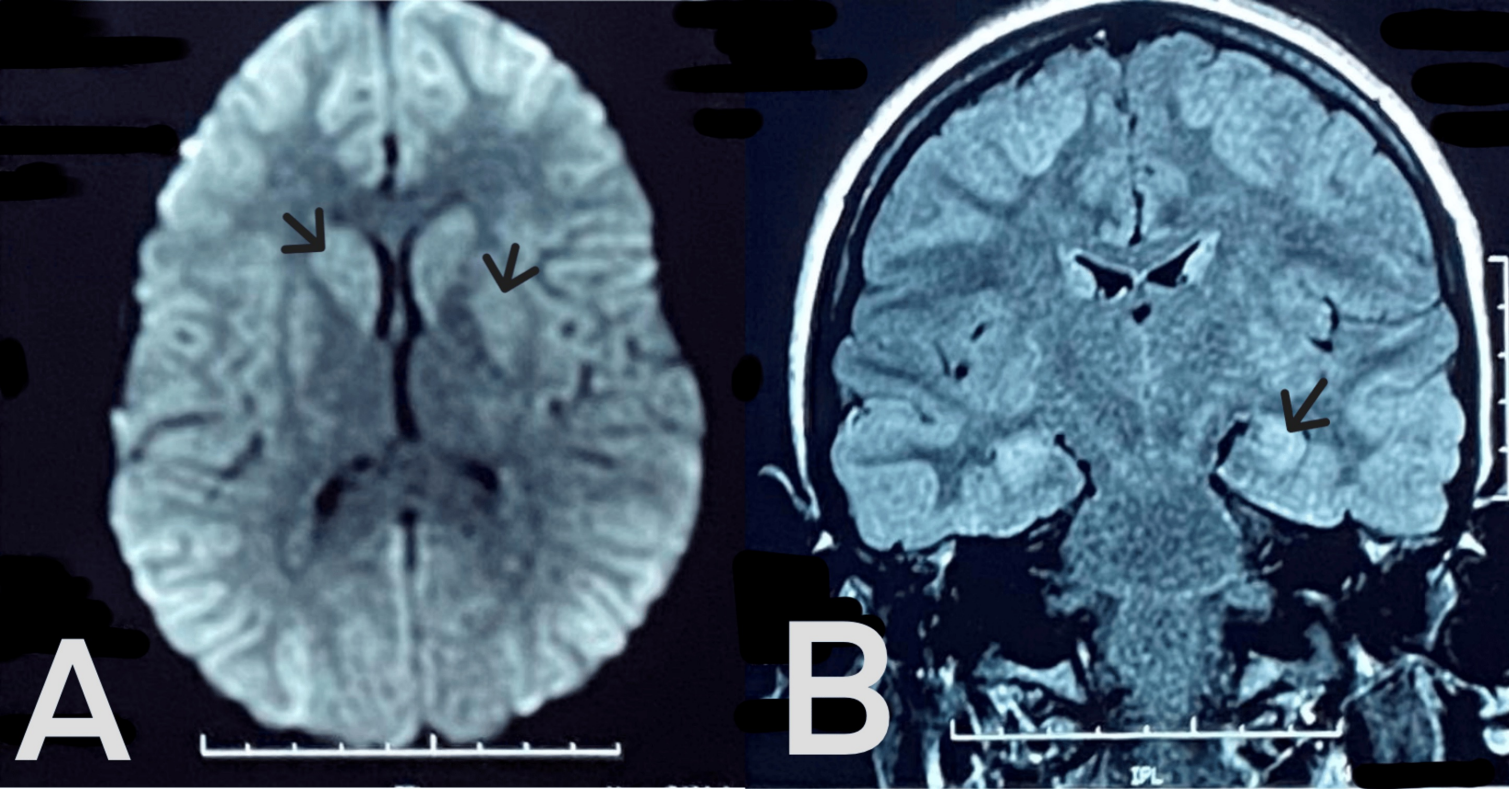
Admission brain magnetic resonance imaging (MRI) (axial and coronal view). (A) Restriction in diffusion sequence on bilateral temporal, occipital, frontal, putamen, and caudate nucleus. (B) Bilateral symmetrical abnormal signal intensity involving the mesial temporal lobe, occipital, and frontal lobes, showing high signal intensity (red arrow) on T2 Fluid-Attenuated Inversion Recovery (FLAIR).
